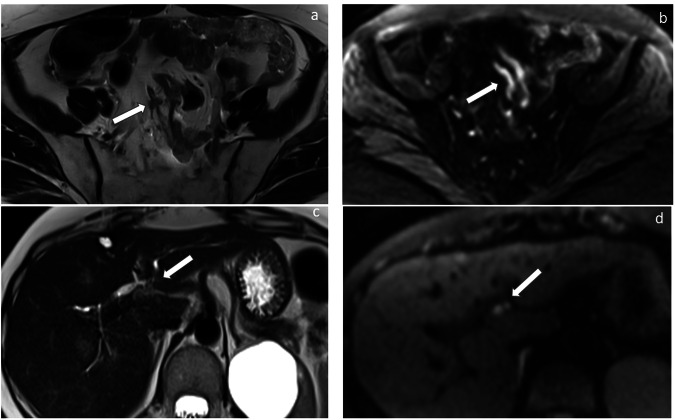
Abdominal MR images showing infiltration of the small bowel mesentery as hypointense thickening of the mesenteric surface on T2w images (a) and as an area with restricted diffusion on DWI MRI images (b); subtle infiltration of the hepatic hilum on axial T2-weighted (c) and high *b*-Value DWI MRI images (d). MRI can be used as a complement to CT as a problem-solving tool, like evaluating the extent of serosal and mesenteric involvement or bowel involvement
